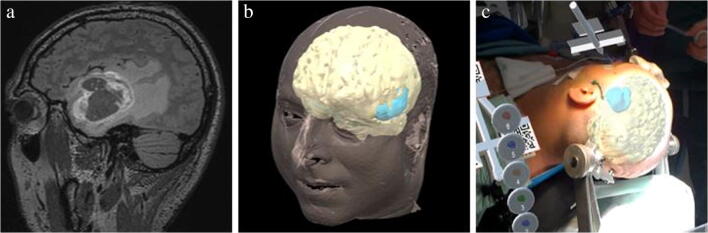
(a) MRI-image of GBM, sagittal view (b) Segmentation (c) Holograms after registration as seen through the HoloLens
